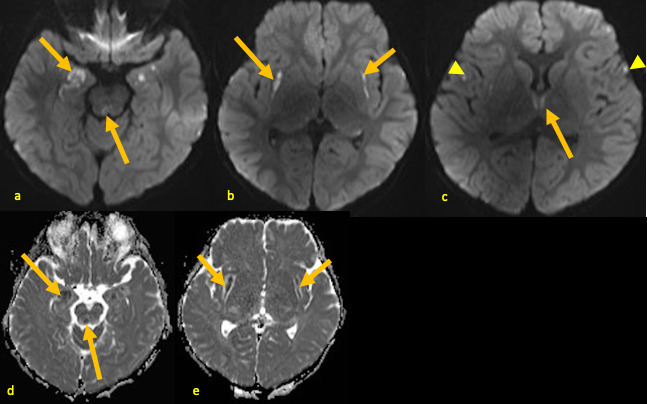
A 25-month-old male with AHNE. Brain MRI obtained during his first presentation for AHNE: axial DWI (a–c) and ADC maps (d, e) demonstrating patchy (arrows) hyperintense DWI signal abnormalities and corresponding low ADC values in medial temporal lobes, periaqueductal regions, medial thalami, and subinsular areas consistent with acute necrosis. Additional punctate foci of diffusion restriction are also noted in frontal cortices (arrowheads), likely postictal changes. ADC, apparent diffusion coefficient; AHNE, acute hemorrhagic necrotizing encephalopathy; DWI, diffusion-weighted image.
